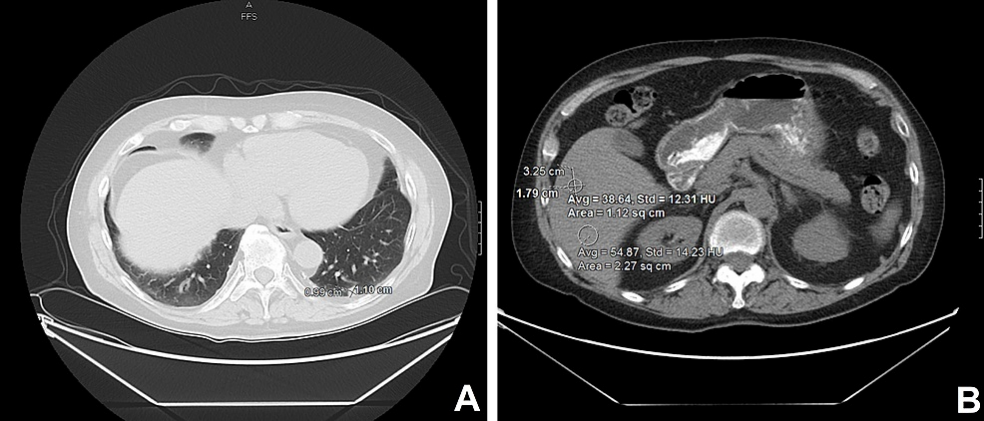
Axial CECT scan of the chest, abdomen, and pelvis at the time of consultation. The images show (A) a 1.0 × 1.1 cm non-calcified left lower lobe pulmonary nodule and (B) a 1.8 × 3.3 cm right liver lobe geographic hypodense region consistent with metastasis. CECT: contrast-enhanced computed tomography
